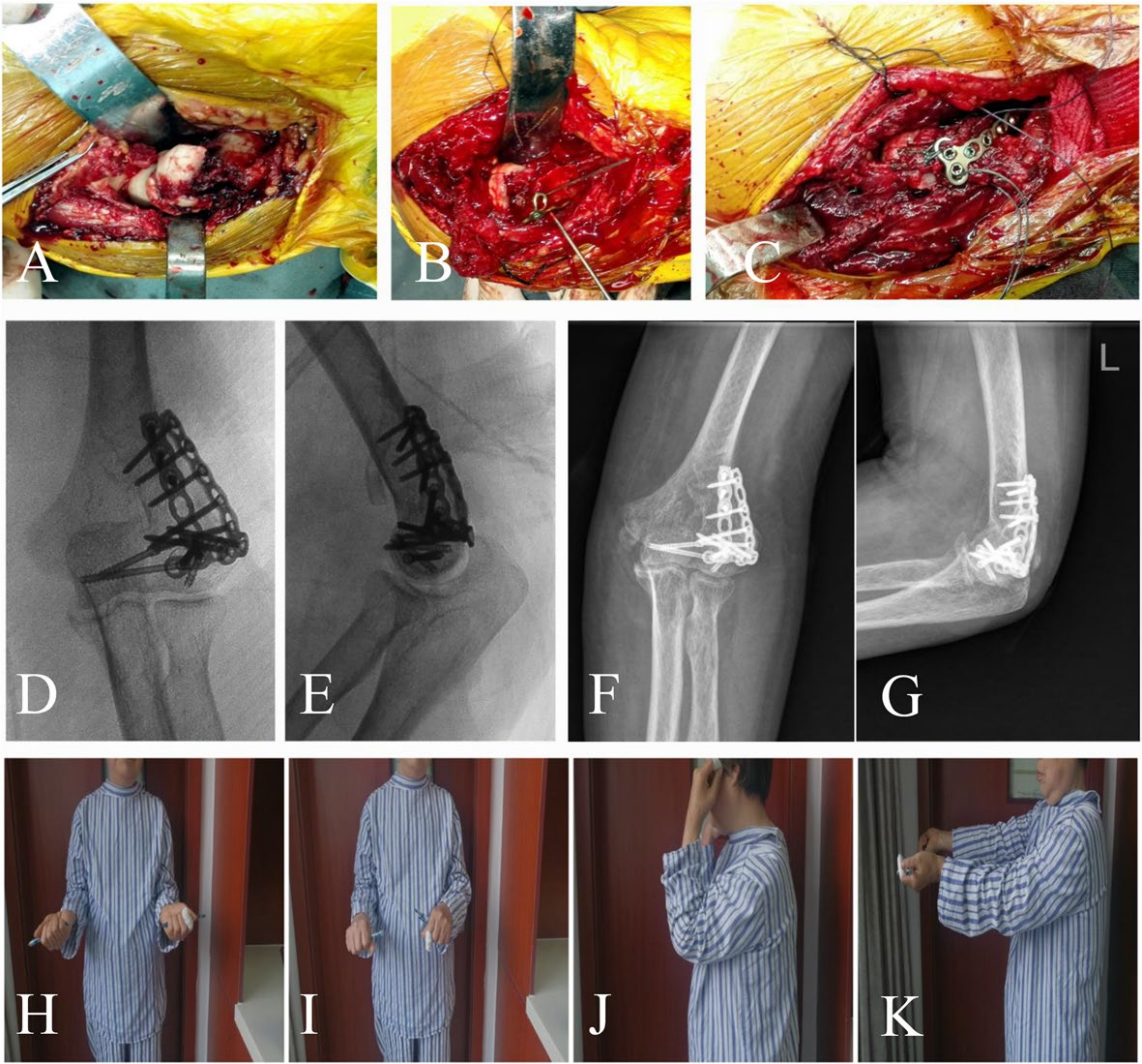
Surgical exposure and fixation of the same patient as in Fig. 1. A A type IIIB fracture is exposed by the extensive lateral approach. The epicondylar fragment with the lateral ulnar collateral ligament is reflected distally to enhance exposure. B Reconstruction of the lateral column is performed with a 2.0 mm metacarpal locking plate. A tricortical iliac crest bone autograft is used to bridge the bone defect. C The lateral ulnar collateral ligament is repaired to its origin using a figure-of-eight tension band wire technique. D, E Intraoperative radiological outcome. F, G X-ray plain film at 18 months after surgery. H–K Mild limitation of elbow flexion and pronation at 18 months of follow-up; the Mayo Elbow Performance Index is 90 points
